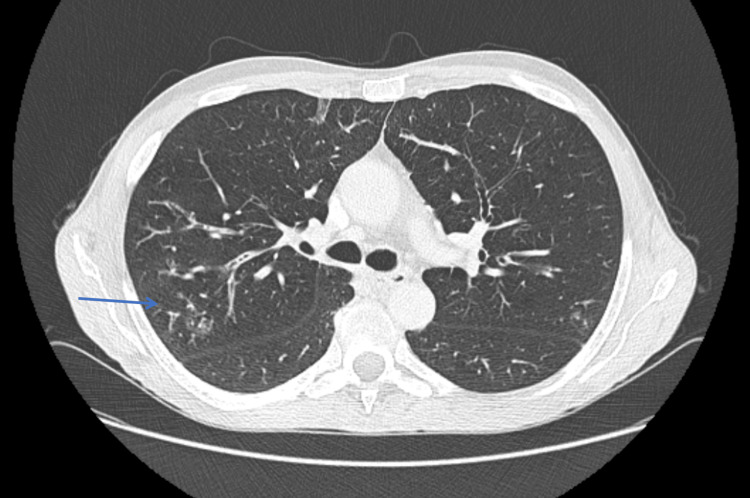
Chest CT in the axial plane showing diffuse tree-in-bud opacities, particularly in the right lung, as indicated by the blue arrow.
